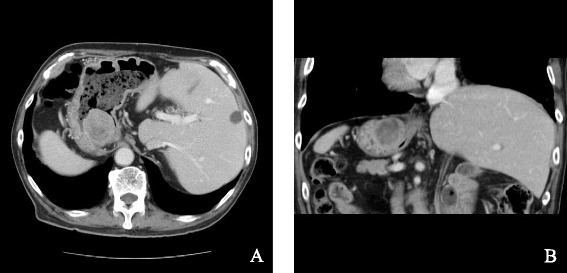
Abdominal CT images. A 45-mm tumor located in the upper area stomach is demonstrated; there is no evidence of distant metastasis. A hepatic cyst is also visible (a). All intra-abdominal organs are inversely positioned (b)
